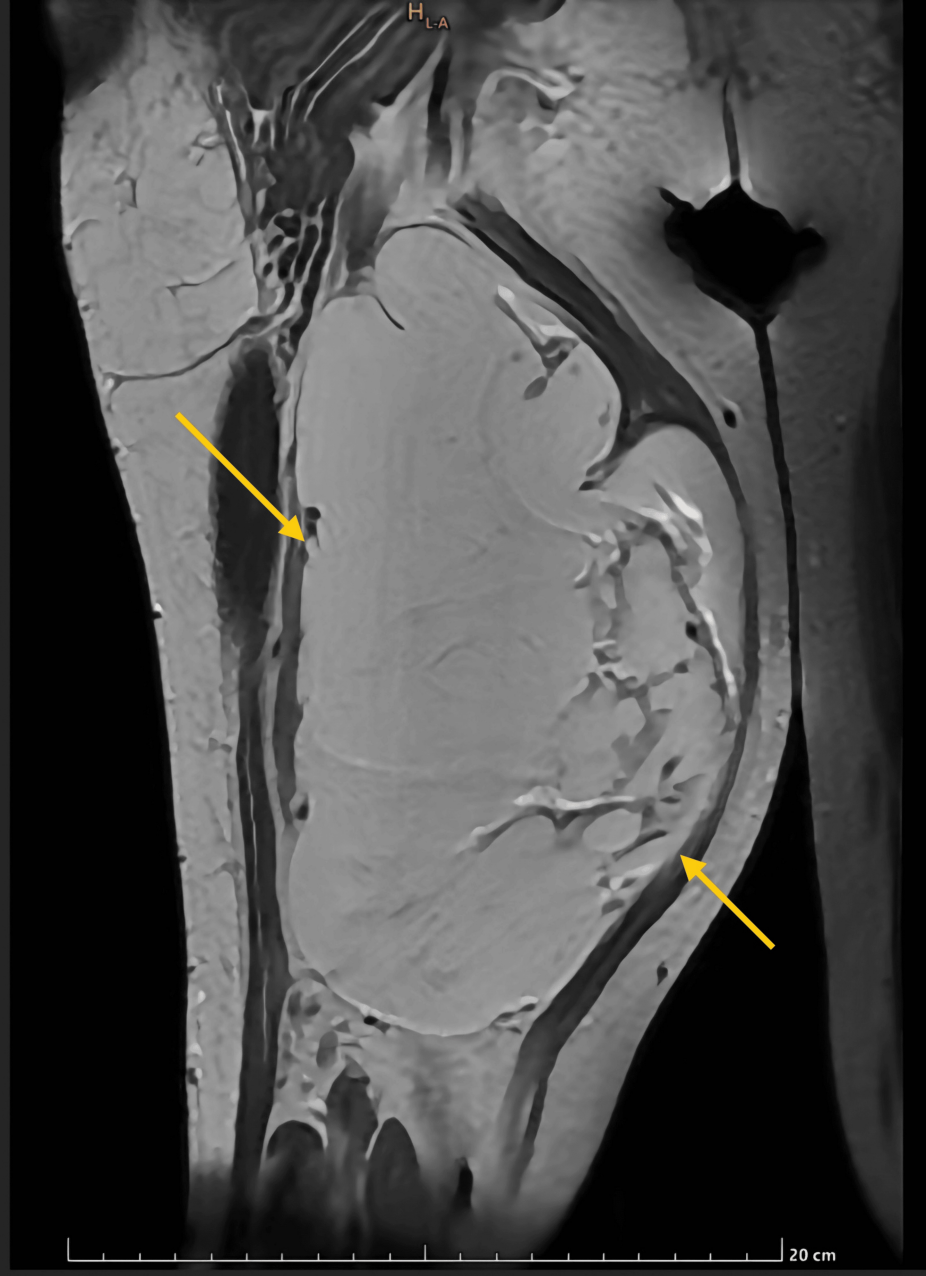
Coronal section of MRI of the right thigh showing the lesion involving the adductor muscles. yellow arrows - depicting the lesion
